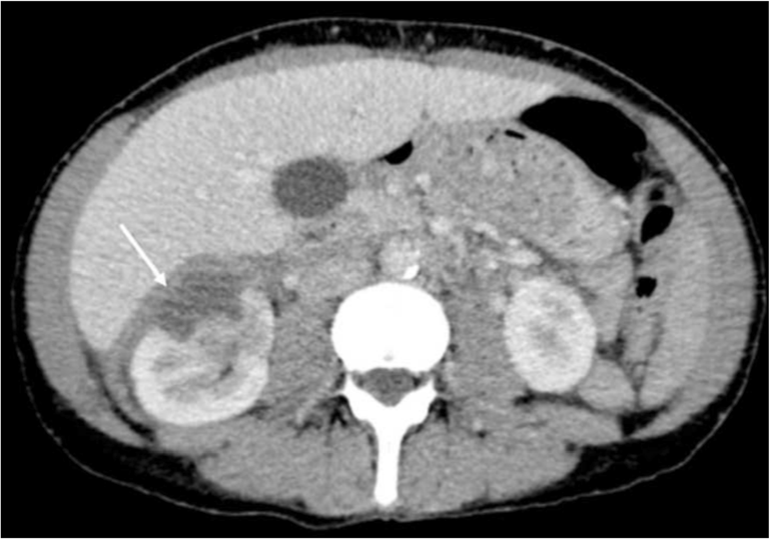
Portal venous CT shows a right renal abscess that has burst into the perinephric space (*arrow*), best appreciated as the soft tissue density interposed between the kidney and adjacent liver
